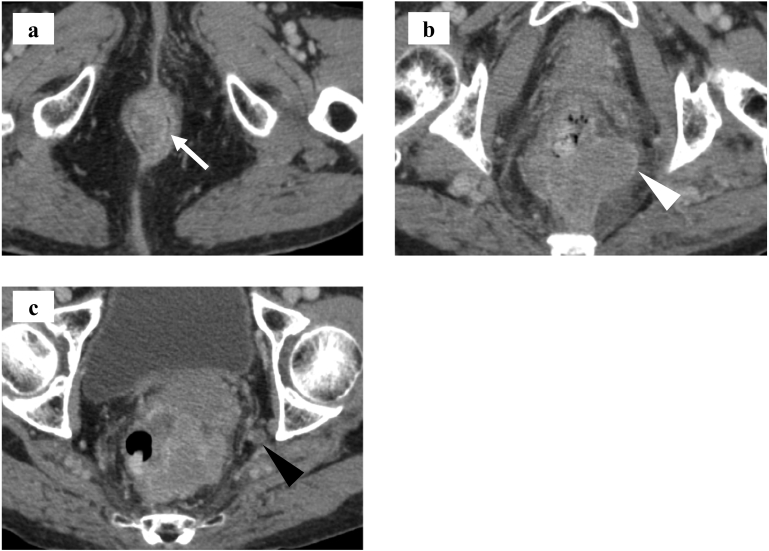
(a) Enhanced computed tomography demonstrated two polypoid tumors with a maximum diameter of 38 in the lower rectum (white arrowhead). (b) A metastatic lymph node of 50 mm in diameter was found in the mesorectum of the upper rectum. (c) A lymph node of 8 mm in diameter in the left internal iliac region (black arrowhead).
